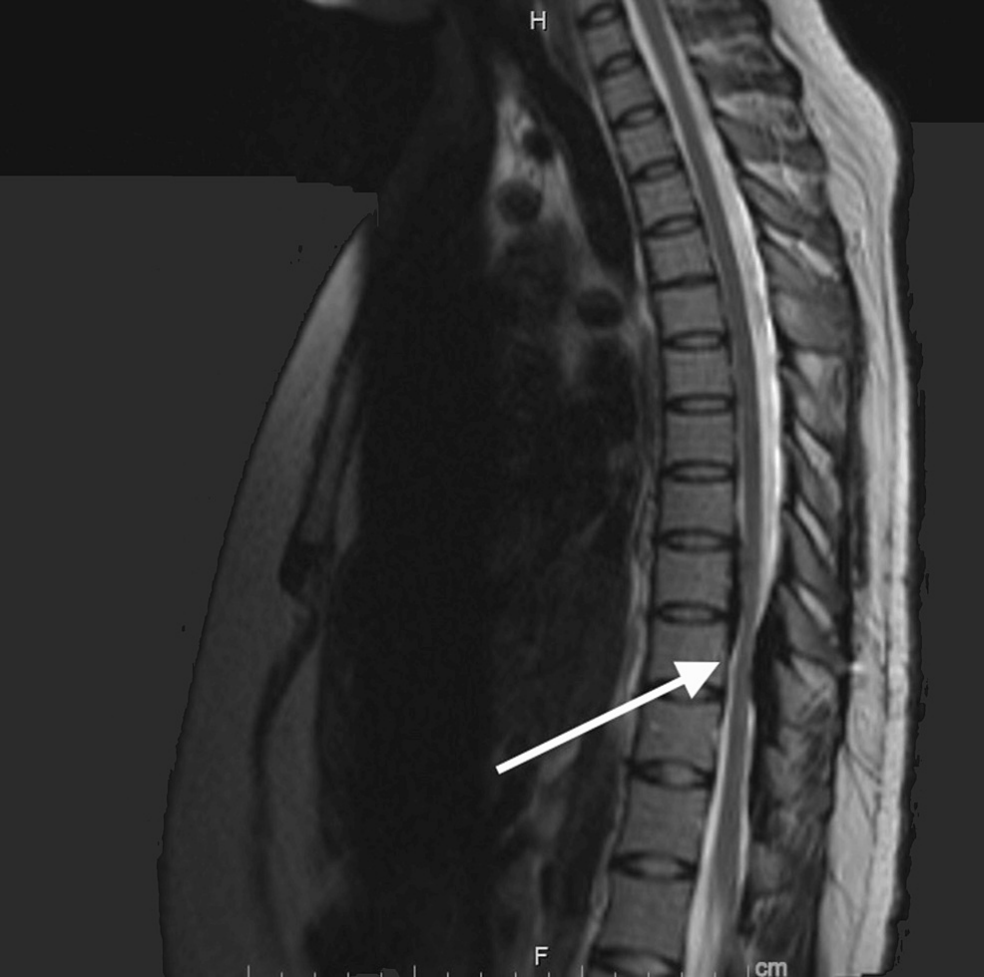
A follow-up magnetic resonance imaging (MRI) of the thoracic spine. A follow-up MRI of the thoracic spine showing relative stability in the size of spinal lesions while receiving rituximab (arrow).
